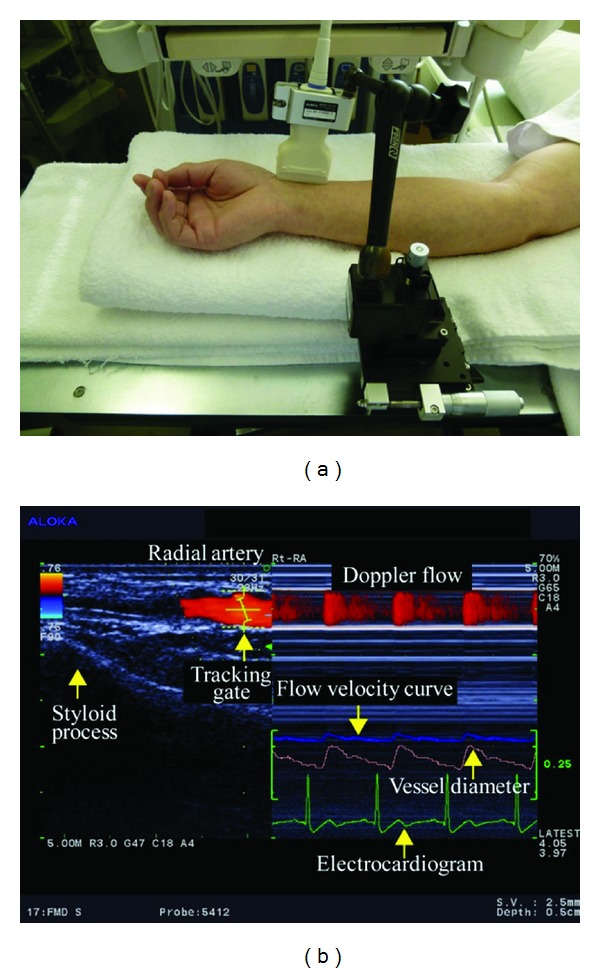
(a) Ultrasound measurement of the radial artery. 13 MHz linear transducer is fixed along radial artery with a special probe holder (MP-PH0001, Aloka Co., Ltd., Tokyo, Japan). (b) Display of CDI. Left: the vessel image and the position of the artery tracking gate. Right: changes in vessel diameter, Doppler flow, and flow velocity as determined by an automated edge-detection device and computer analysis software (e-Tracking system; Aloka Co., Ltd., Tokyo, Japan).
